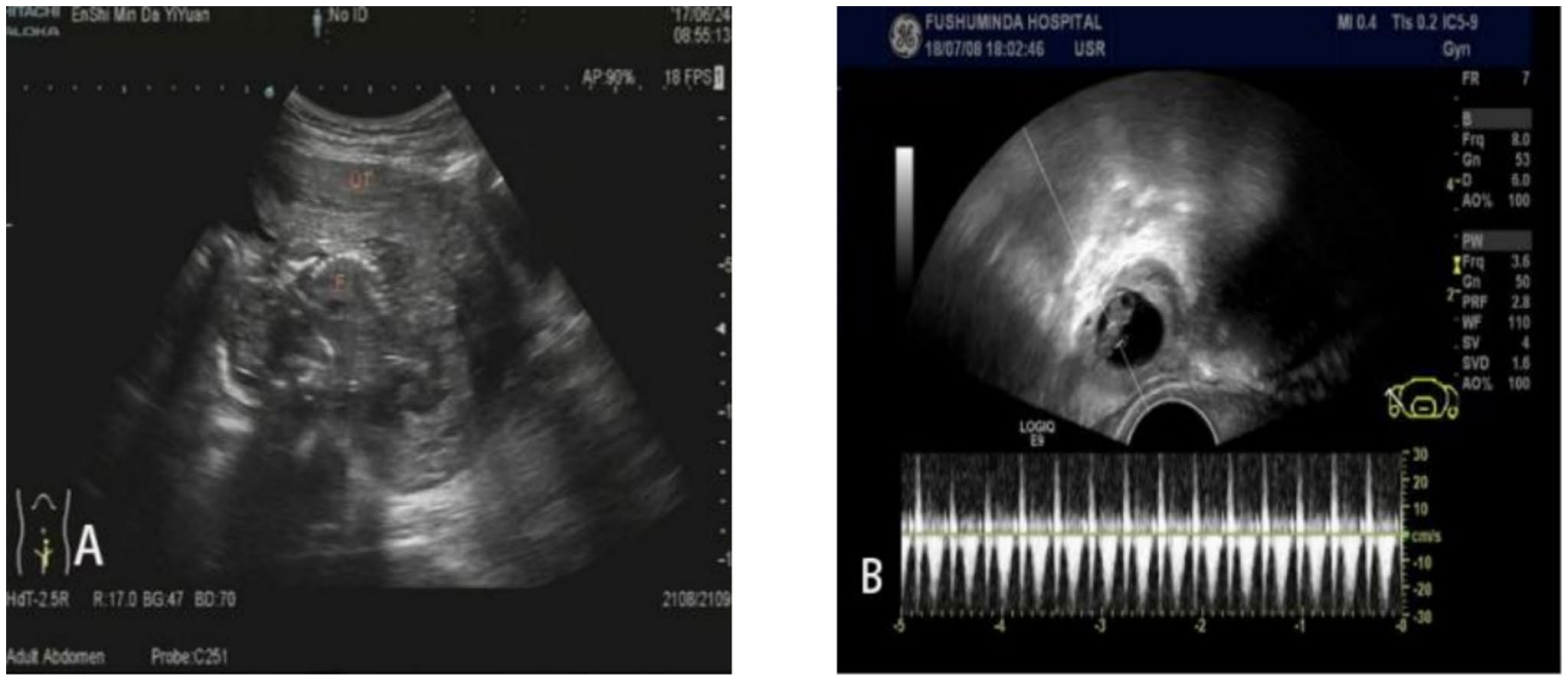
Ultrasonography images of Douglas pouch pregnancy of the fifth patient. The two pictures (A) and (B) show different sections of the lesion area of the fifth patient.
